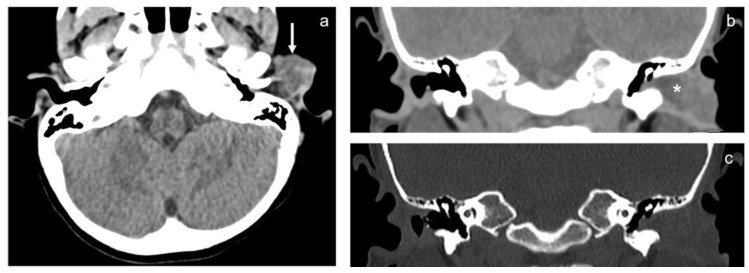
(a) CT axial view. White arrow shows the mass that extends outside the left EAC. (b) and (c) CT coronal view. * shows the mass.
